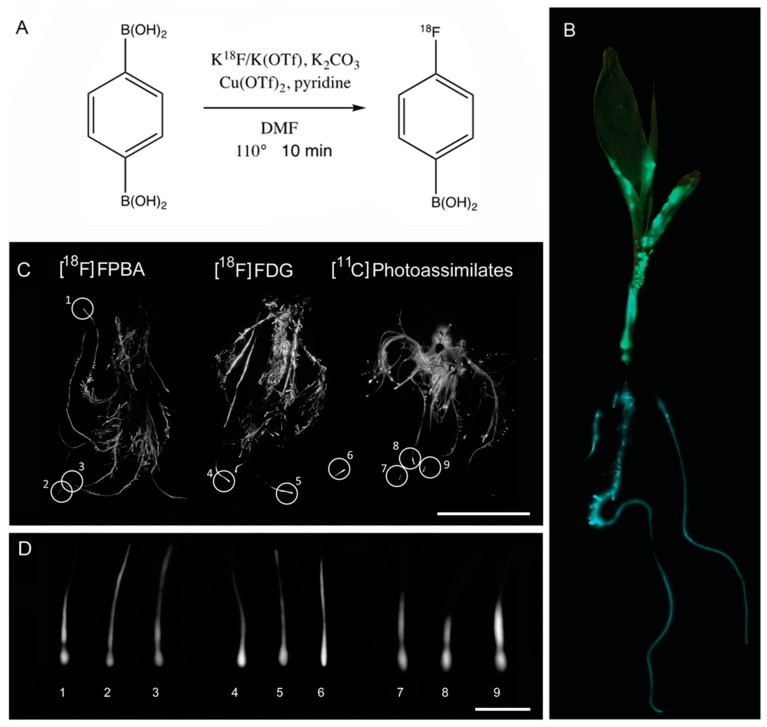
Synthesis and administration of a [^18^F]FPBA radiotracer to maize seedlings (inbred B73). (A) Single vial synthesis of [^18^F]FPBA from precursor 1,4-diboronic acid using a copper catalyst. (B) Radiographic image overlaid on visual image of five-days-old maize seedling after passive hydroponic administration of [^18^F]FPBA radiotracer. (C) Three-weeks-old maize root radiographic images under passive administration of [^18^F]FPBA, [^18^F]FDG, or [^11^C]CO_2_. Regions of interest appearing in circles are shown under greater magnification in panel D. (D) Primary root tips and elongation zones as they appear under radiographic imaging using [^18^F]PBA, [^18^F]FDG, or [^11^C]CO_2_. Scale bar in C = 15 cm and in D = 0.5 cm.
